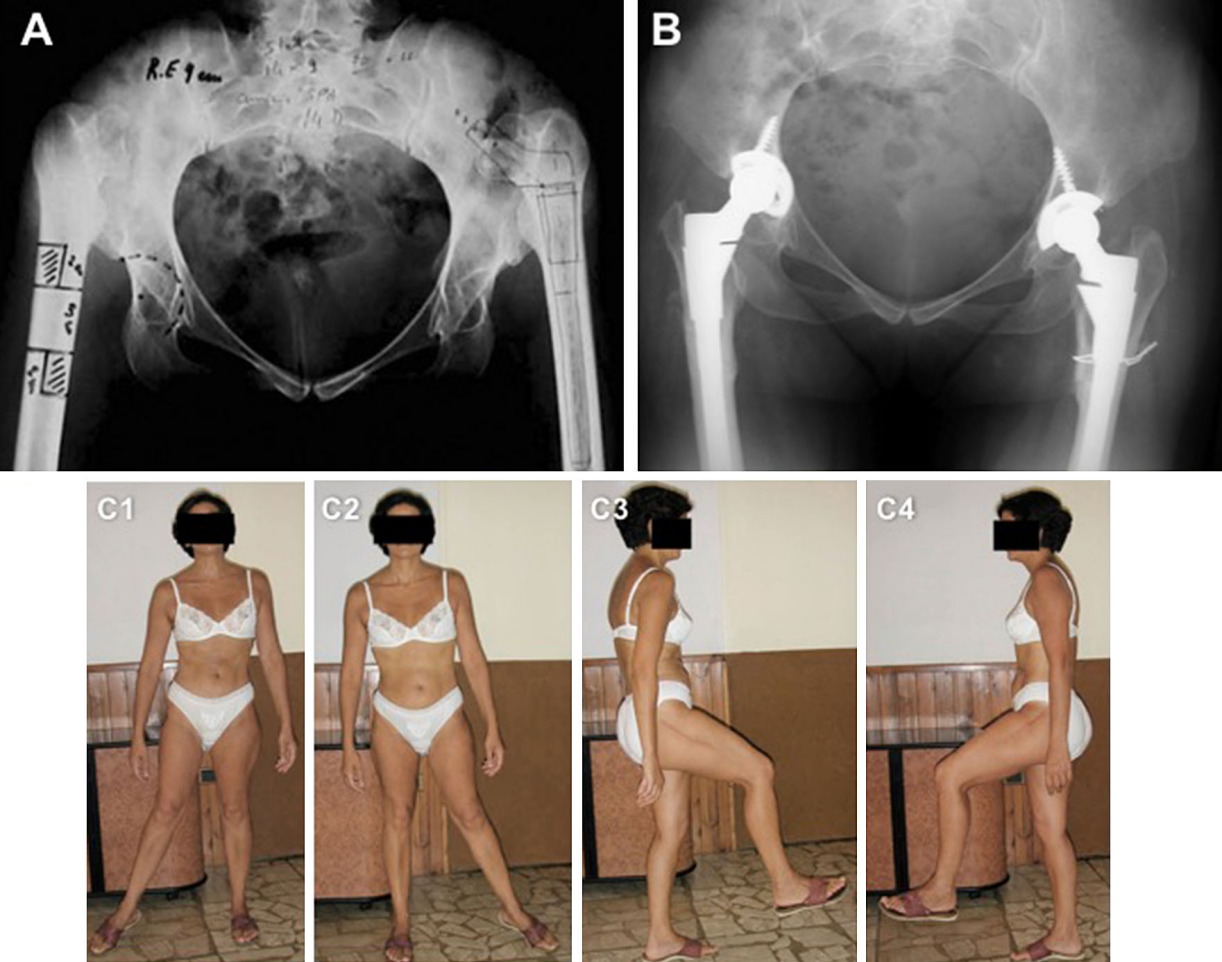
44-year-old female patient with bilateral DDH. a Pre-operative planning with the Z-shaped osteotomy. b X-ray at 2-year follow-up right hip surgery (1-year; *left* hip). Note the intra-op fracture on the *left* hip treated with an additional cerclage. c1–4 Clinical images at 5-year follow-up right hip surgery (4-year; *left* hip)
